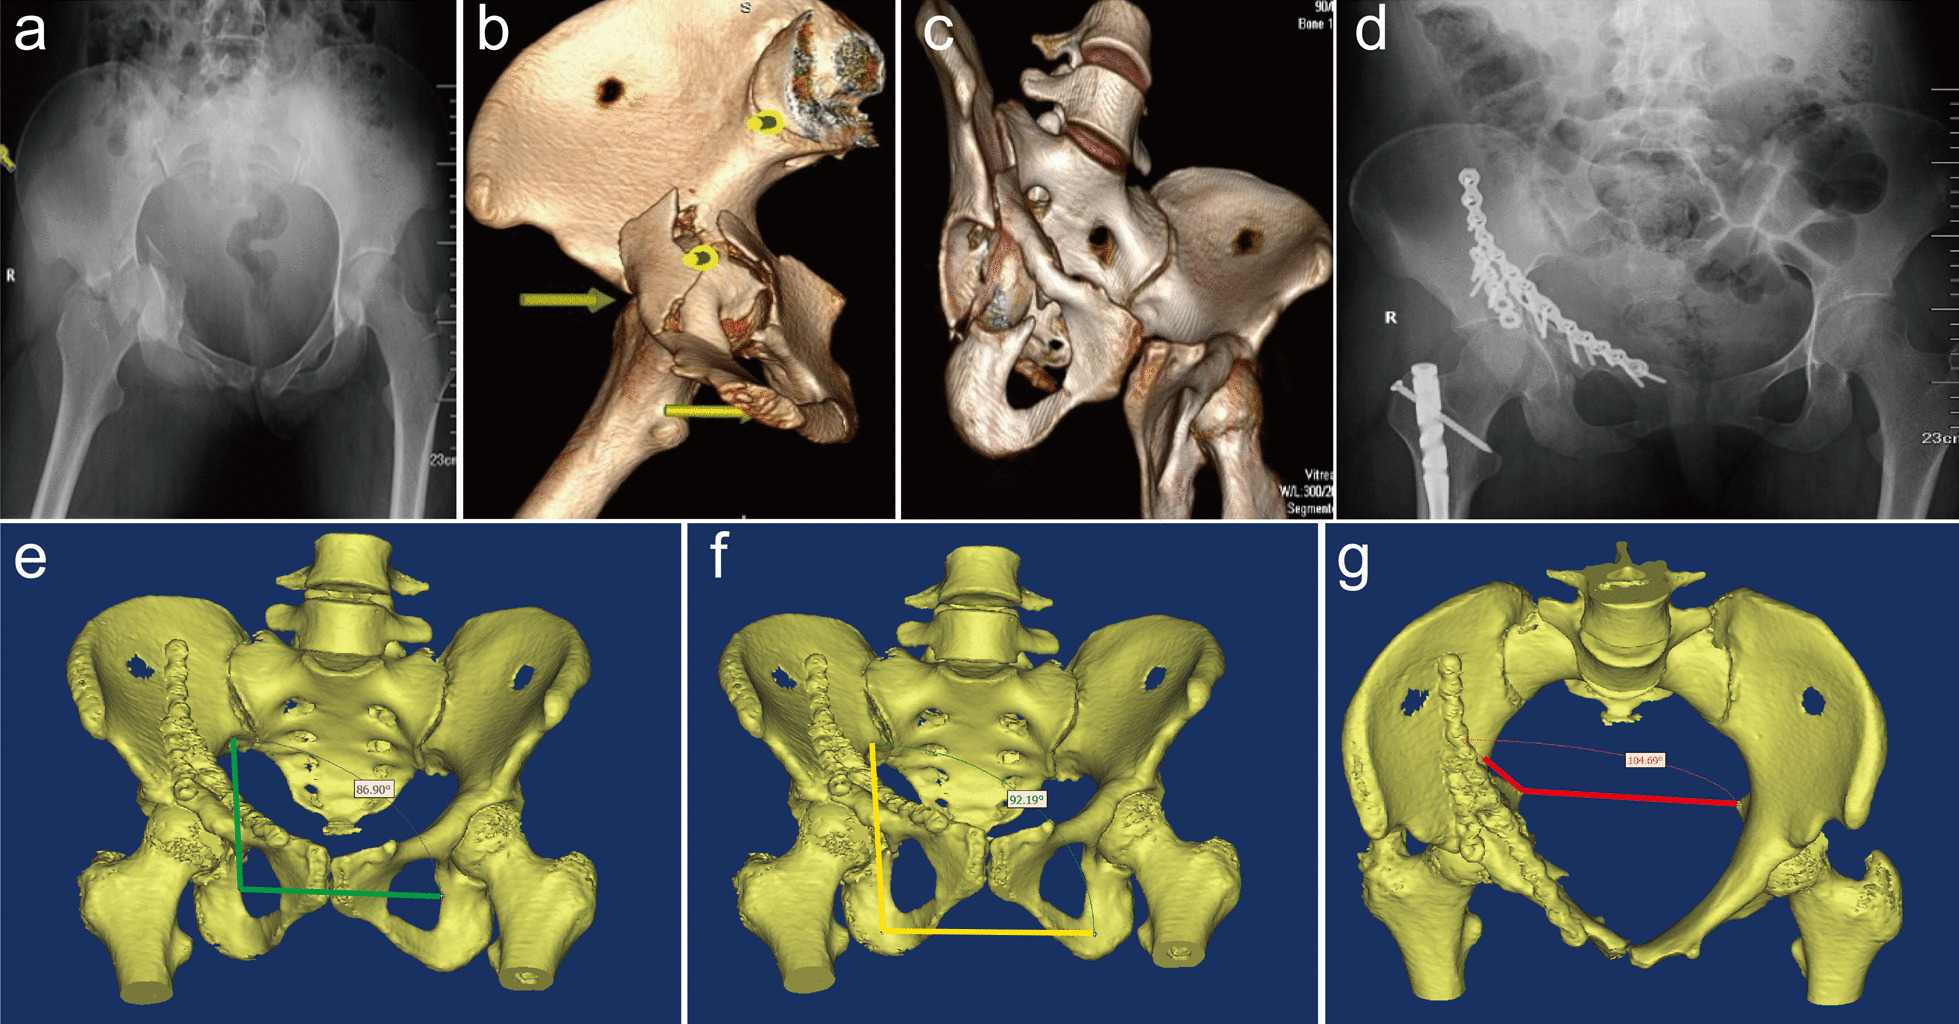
Typical case: A 23-year-old woman presented with T-shaped fracture of the right acetabulum (Letournel-Judet classification). Preoperative X-ray (a) and CT 3d reconstruction (b–c) showed significant fracture displacement of quadrilateral plate. Dynamic anterior plate-screw system for quadrilateral plates (DAPSQ) fixation was performed by using the ilioinguinal approach. Postoperative X-ray (d) showed good fracture reduction. Postoperative 3D CT reconstruction showed residual displacement was less than 1 mm and graded as excellent according to classic Matta criteria. e The anterior inclined angle of quadrilateral plate was 86.90°; f The middle inclined angle of quadrilateral plate was 92.19°; g The posterior inclined angle of quadrilateral plate was 104.69°. All the three inclined angles were not within normal range, and rated as poor according to evaluation criteria of inclined angles. Combined with the classic Matta criteria, the modified Matta criteria of this patient graded as good. The Harris hip score of the last follow-up was 75, graded as fair
